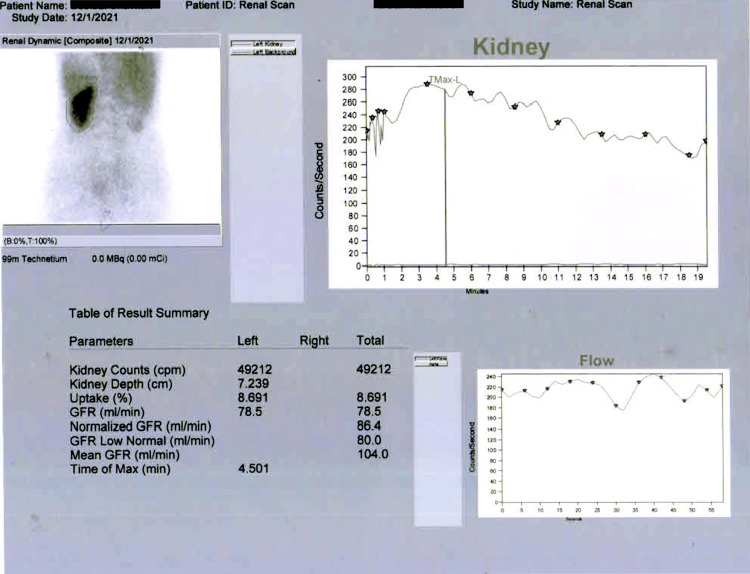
Renal scintigraphy scan showing homogeneous cortical tracer extraction over the left kidney but no significant appreciable cortical tracer extraction or tracer excretion noted throughout the study over the right kidney.
